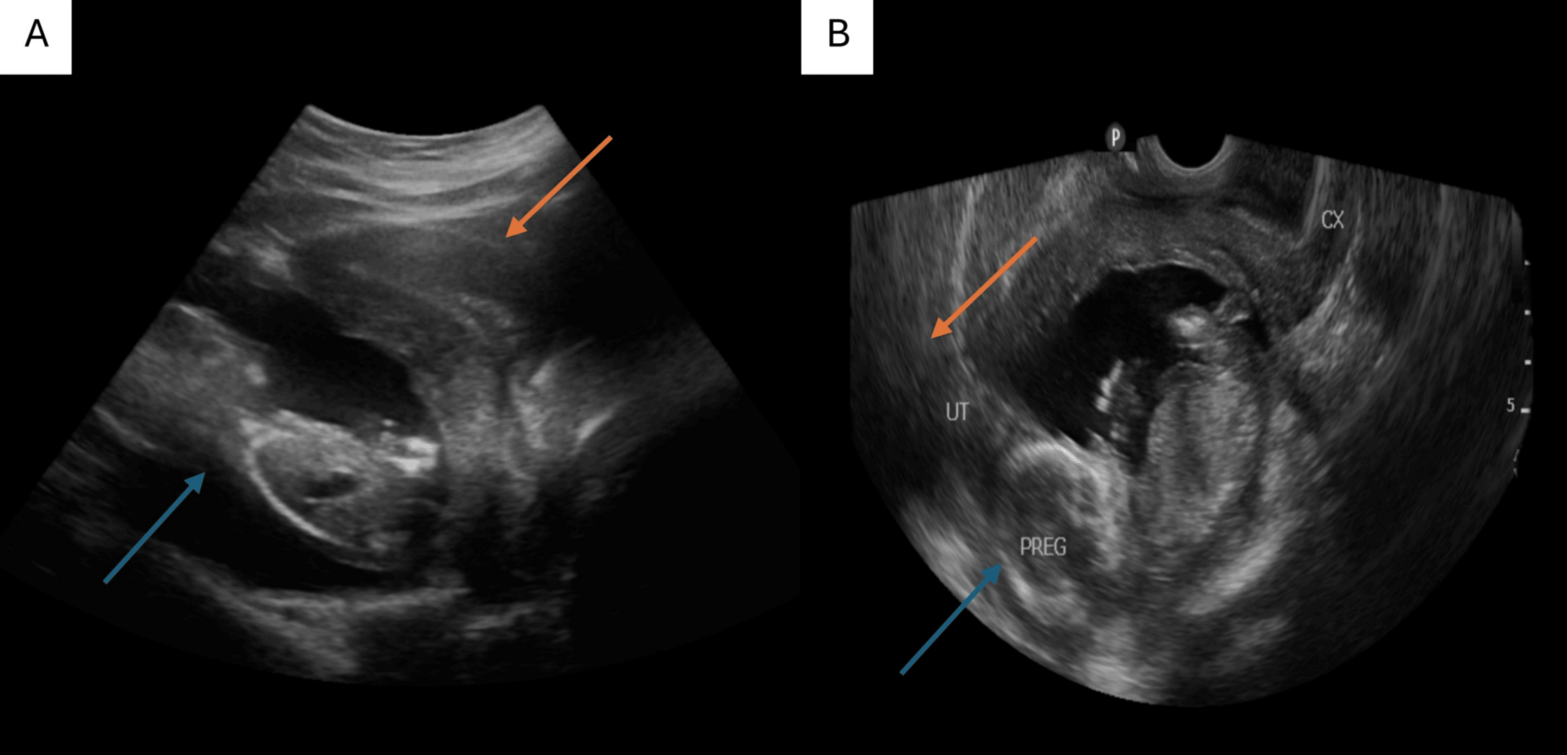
Obstetric ultrasound. Still images (A and B) show an empty uterus (orange arrow) and the fetus located outside the uterus (blue arrows).
